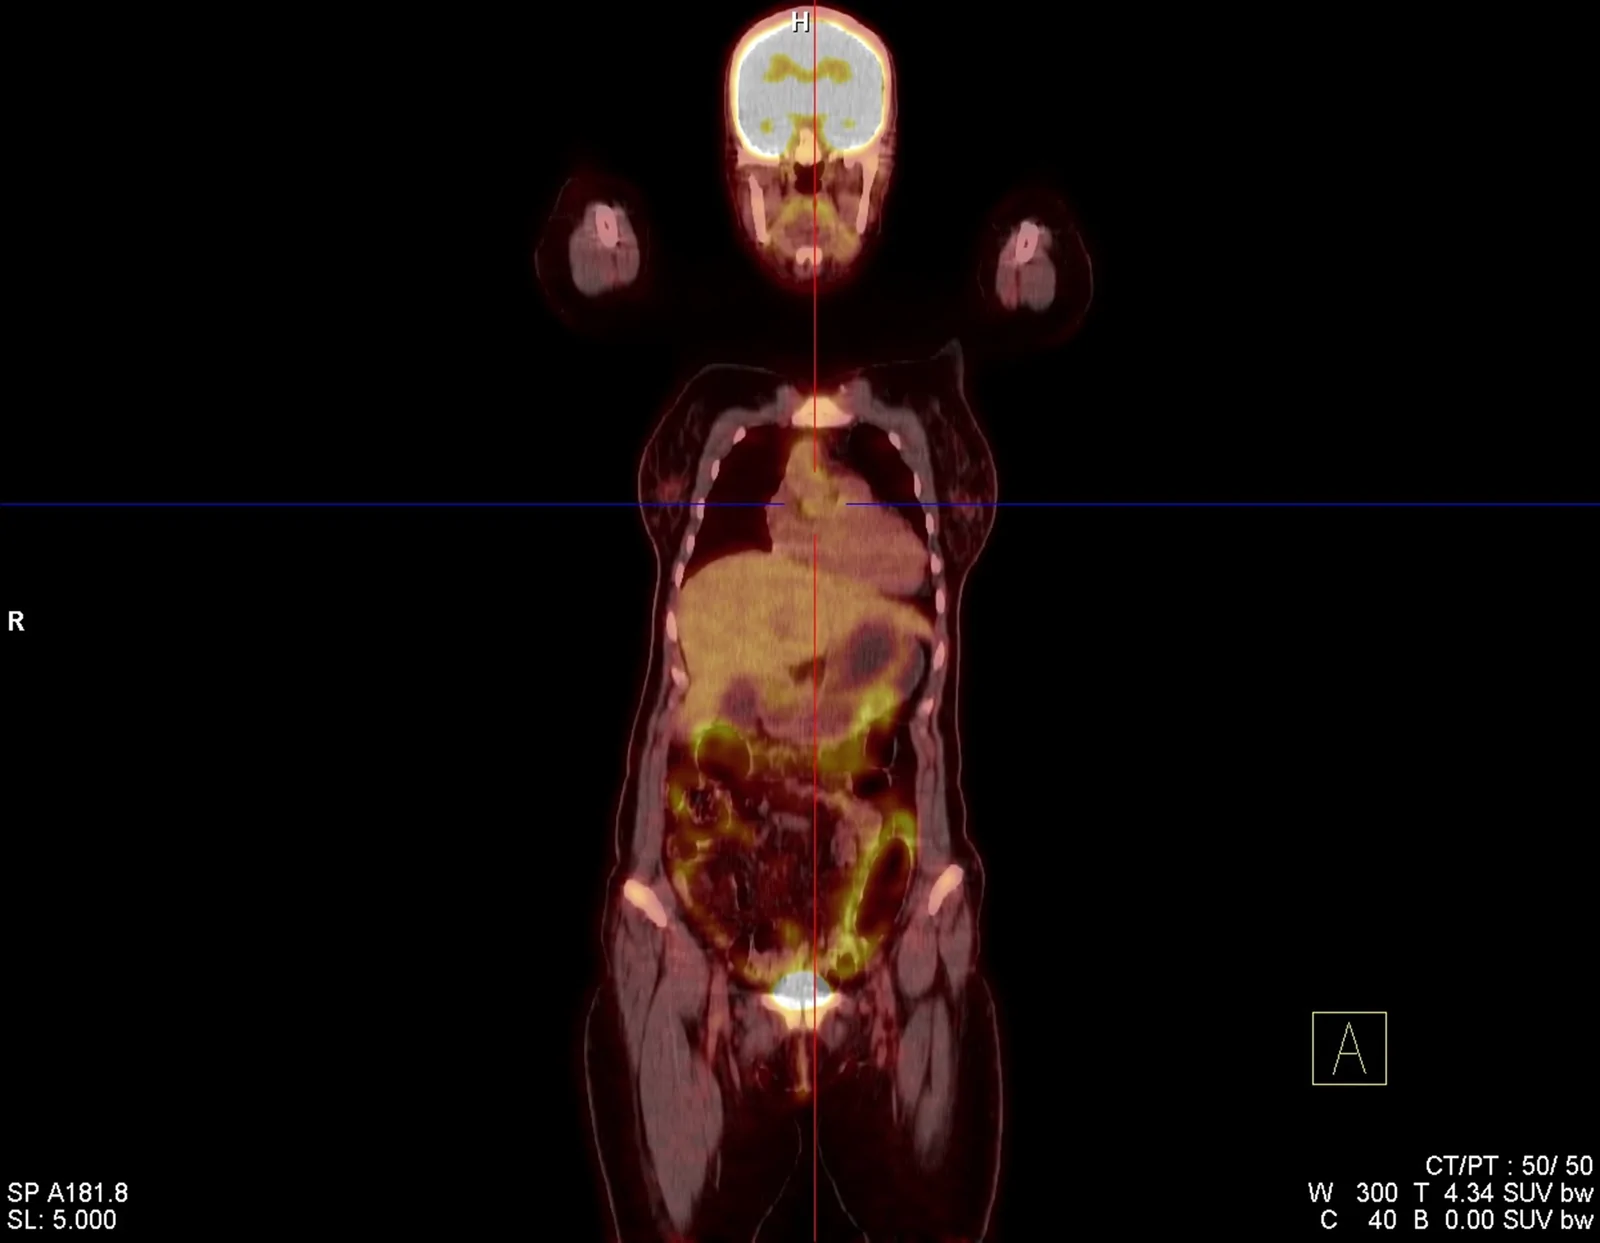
Whole-body ¹⁸F-FDG PET scan (coronal view) demonstrating pathologically increased FDG uptake along the aortic wall and its supra-aortic branches
